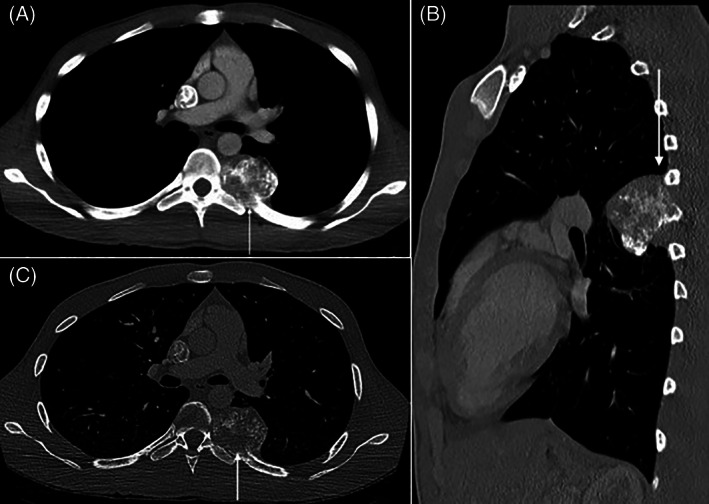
Initial axial contrast–enhanced chest CT mediastinal window (A), axial (B) and sagittal l bone window (C) images demonstrates a 5 cm mass arising from posterior left seventh rib containing cloud like calcification (white arrow) without spinal canal or neural foraminal extension
